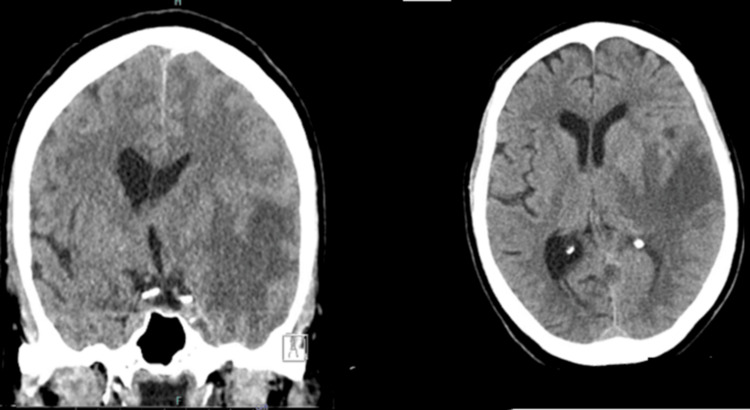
CT head without contrast on admission Findings of confluent area of edema with relative sparing of the cortex, predominantly involving the left temporal with extension into surroundings with mass effect on the left lateral ventricle with risk for herniation.
